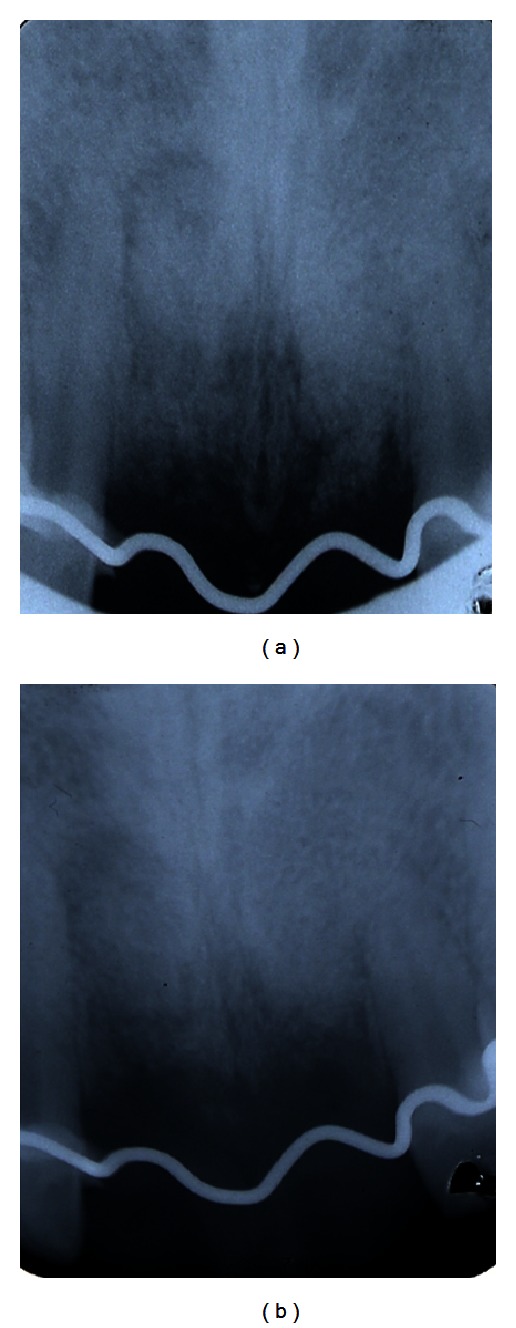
A periapical radiograph was taken at 3 (a) and 6 months (b) from the socket preservation. After 6 months, a limited bone loss was showed related to the steel wire of the interim prosthesis.
